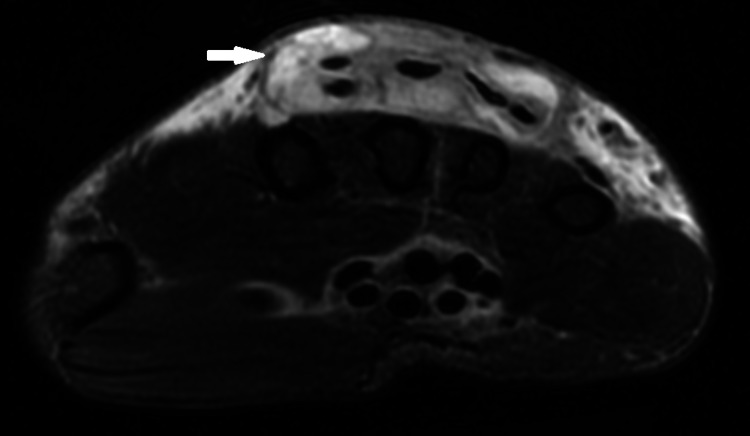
MRI of right upper extremity without contrast The image is showing abnormal tissue and edema within the sheath of the extensor digitorum, intermediate to high signal in T2-weighted images, consistent with prominent tenosynovitis.
